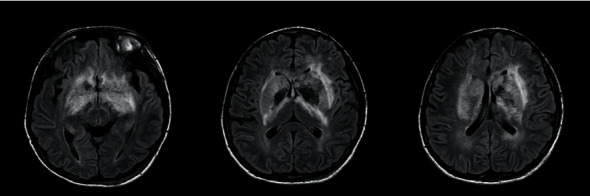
T2-FLAIR image, an axial section at basal ganglia.
